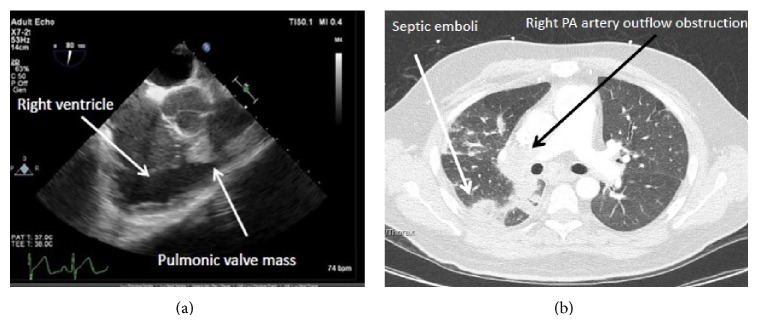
(a) Transesophageal echocardiogram demonstrating a large mass in the right ventricular outflow tract during diastole. (b) CT angiography of the chest showing a right PA artery outflow obstruction and septic pulmonary emboli.
